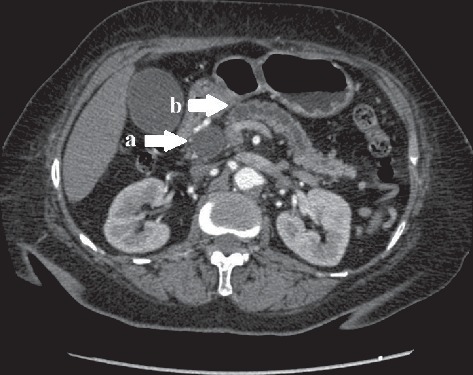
Computed tomography scan showing an ill-defined, hypodense mass at the pancreatic head, causing upstream dilatation of the common bile duct (a) and pancreatic duct (b) compatible with the double duct sign.
